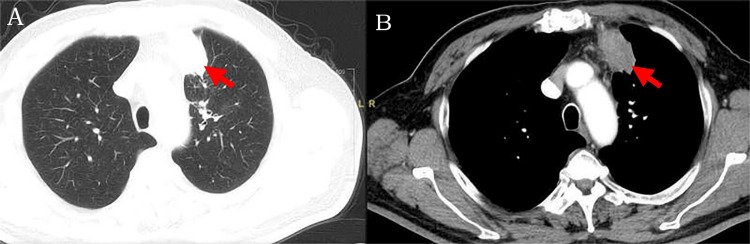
(A) CT lung window. (B) CT mediastinal window. The contrast-enhanced CT scan of the patient's chest revealed a 50.0 × 39.2 mm soft tissue density shadow in the left upper apical lobe, showing notable enhancement. This finding is highlighted in (A) and (B), indicated by red arrows.
